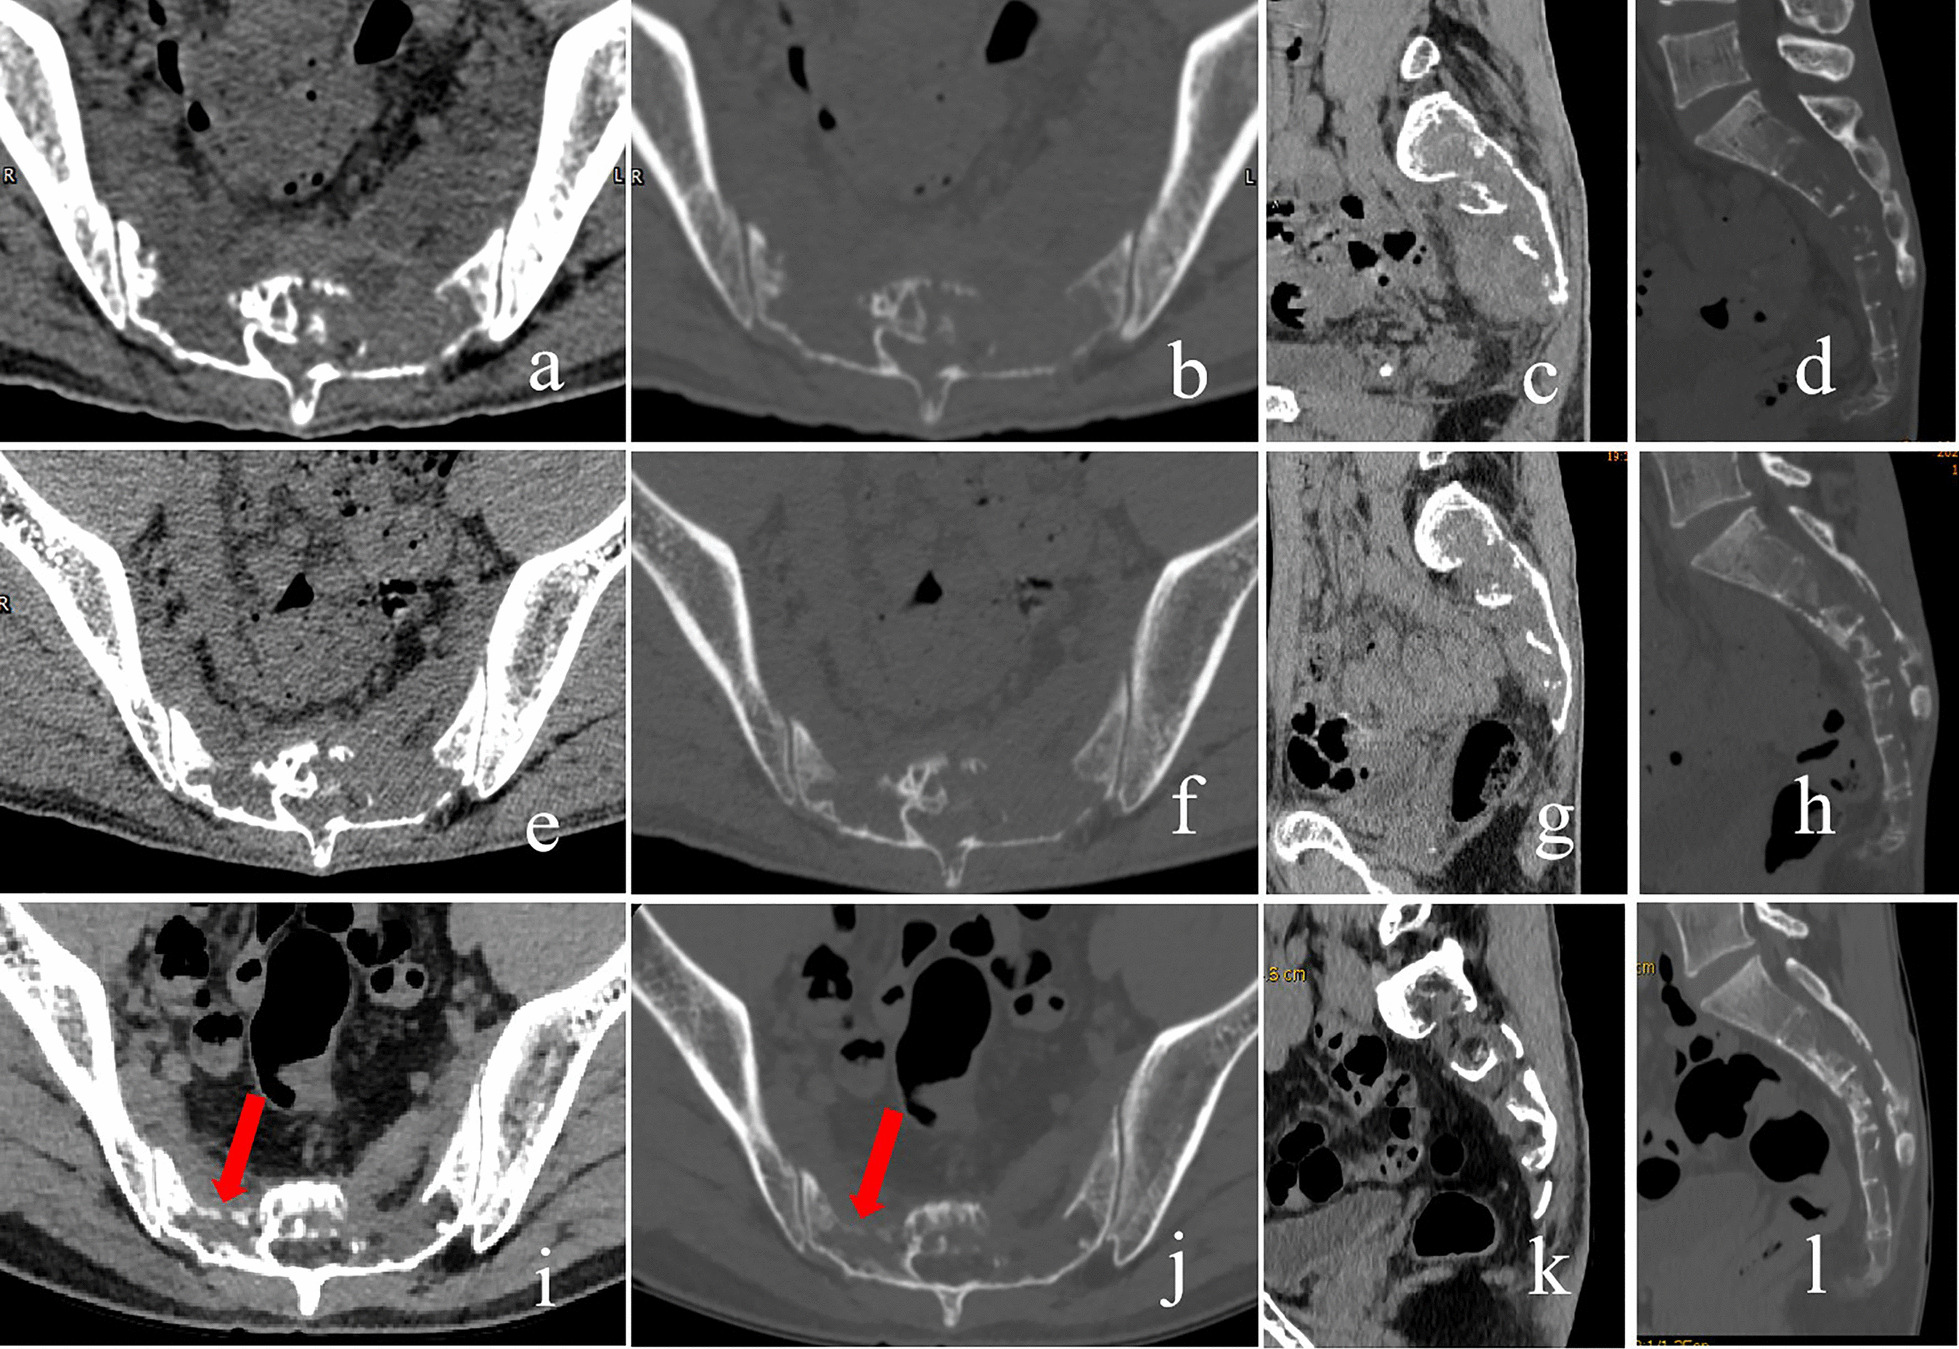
Postoperative computed tomography (CT) (sacrum). One-month postoperative CT (a transversal soft tissue window; b transversal bone window; c sagittal soft tissue window; d sagittal bone window) -abscess became smaller, and bone defect lesions show no changes. Three-month post-operative CT (e transversal soft tissue window; f transversal bone window; g sagittal soft tissue window; h sagittal bone window): no abscess, bone defect lesions became smaller. Six-month post-operative CT (i transversal soft tissue window; j transversal bone window; k sagittal soft tissue window; l sagittal bone window): new bone on edge of bone defect lesions (red arrows) and no relapse
